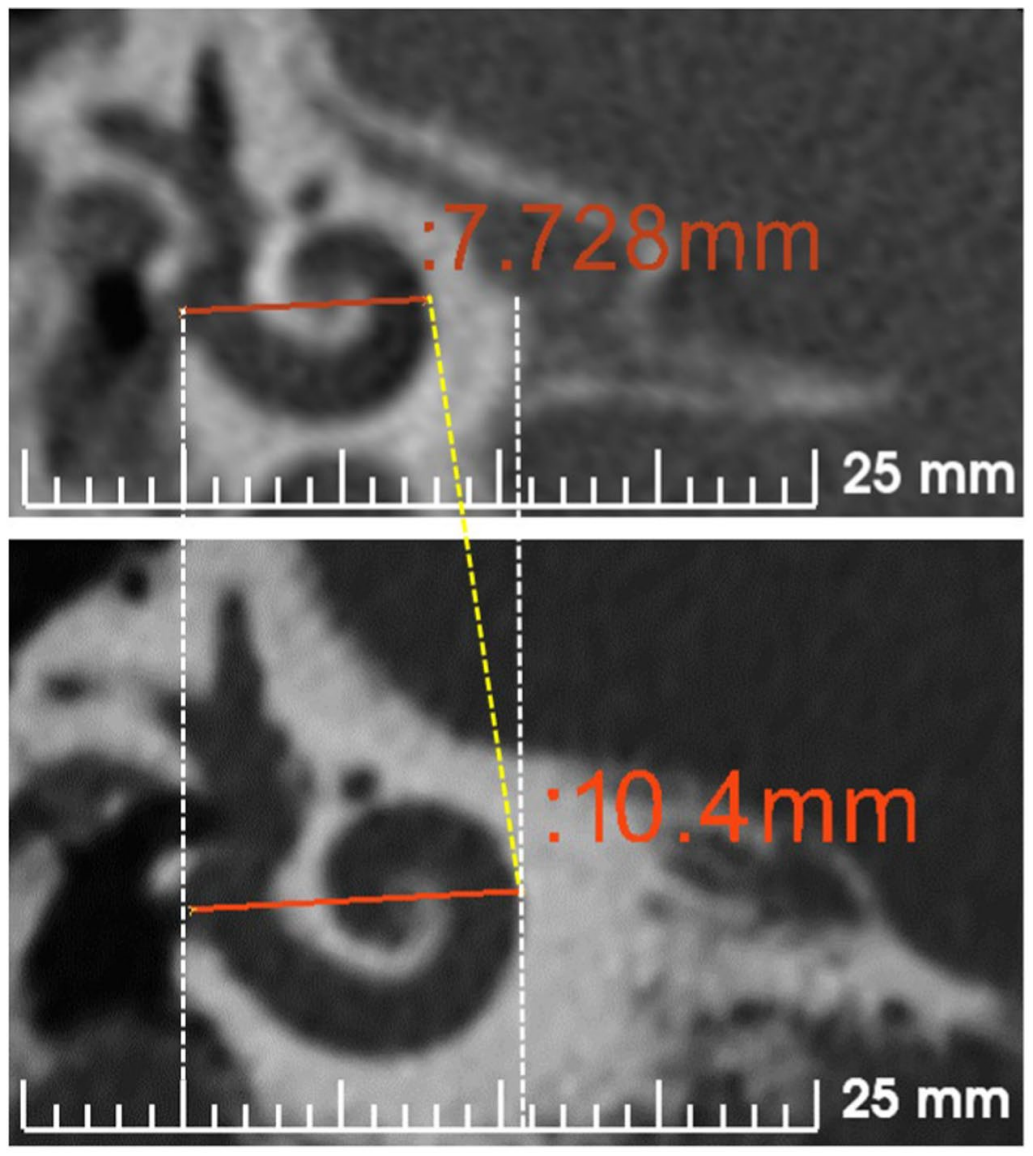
Cochlear size measured according to *A*-value in the oblique coronal view. CT scans showing the cochleae of different sizes are from anonymized subjects from St. Petersburg ENT and Speech Research Institute, Russia (33).
